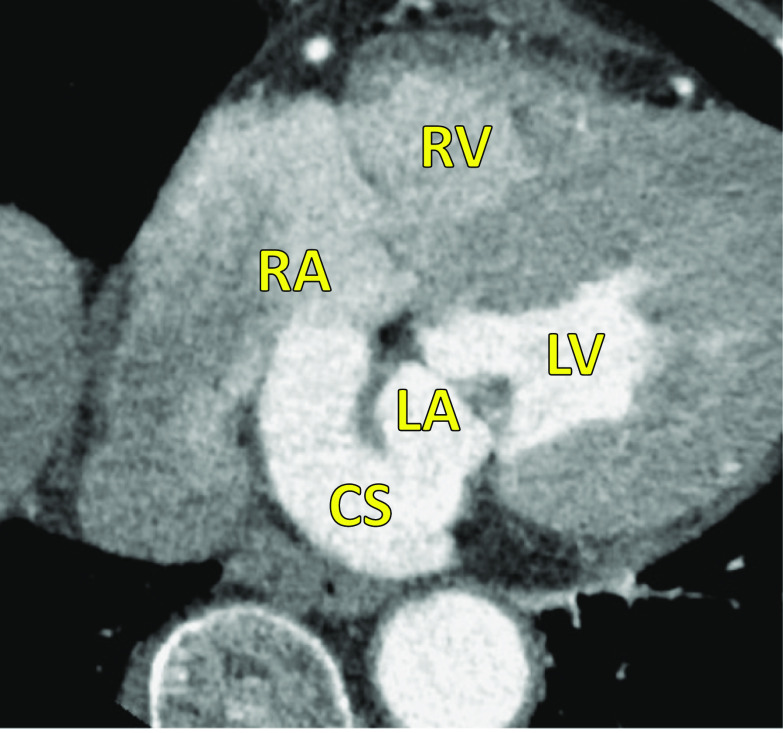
Preoperative cardiac computed tomography. Preoperative cardiac computed tomography showed extensive communication between the right and left atria caused by partial absence of the roof of the coronary sinus. *LA* left atrium, *LV* left ventricle, *RA* right atrium, *RV* right ventricle, *CS* coronary sinus
